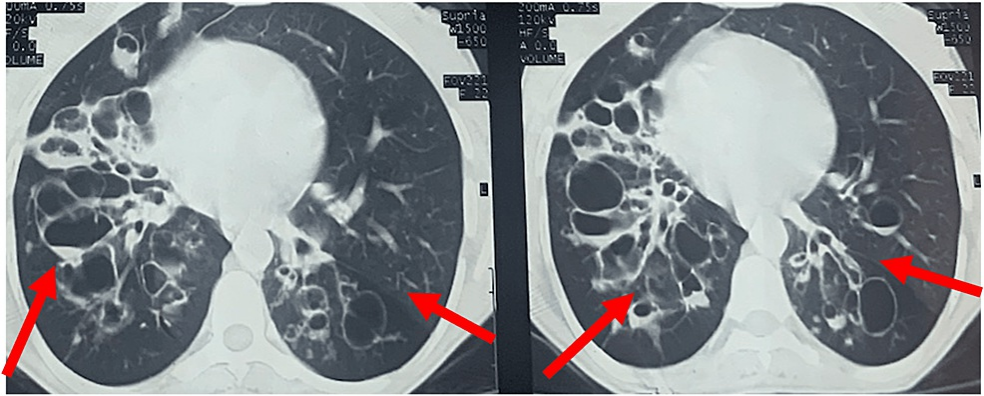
CT thorax of bilateral cystic bronchiectasis (red arrow) middle and lower zone
